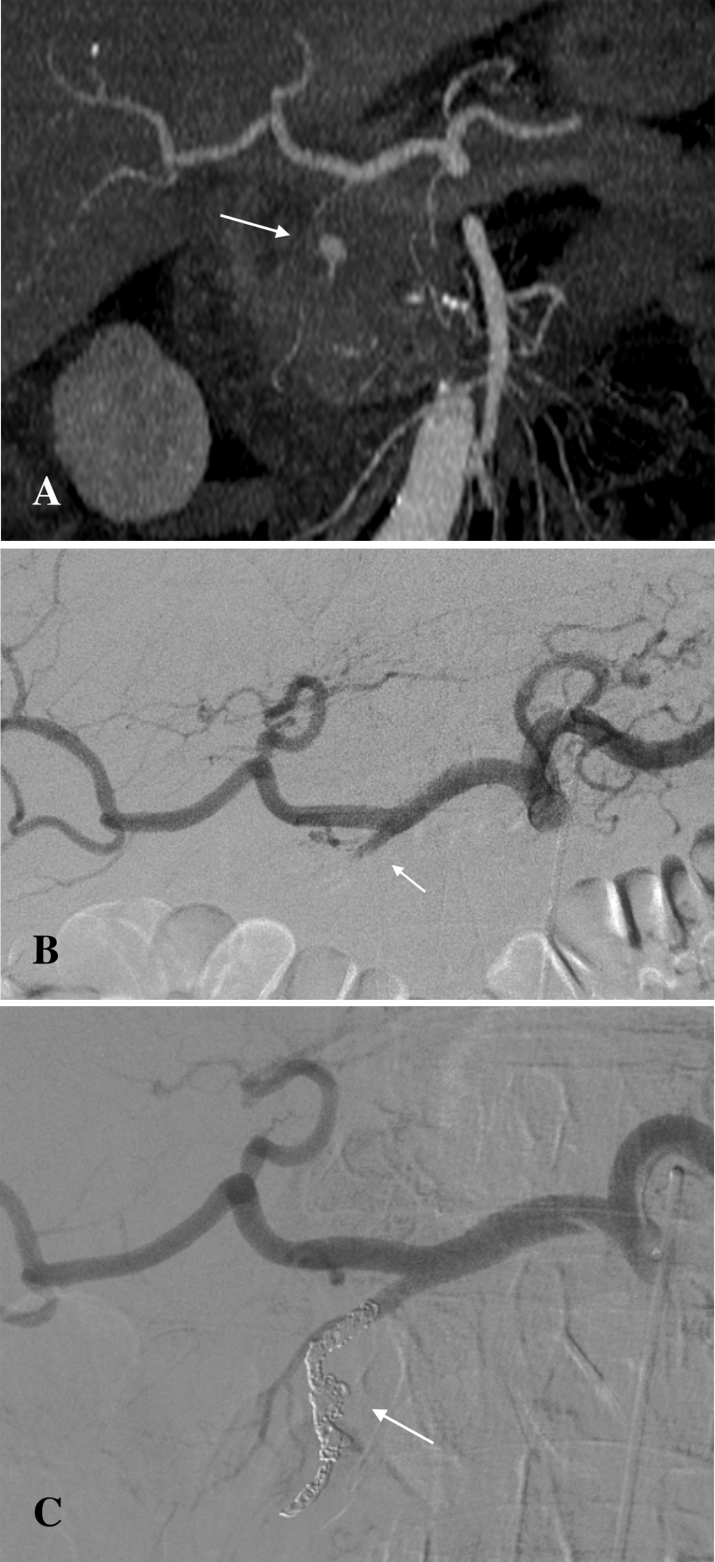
Pseudoaneurysm of the gastroduodenal artery pre- and post-coil embolization. (A) Pseudoaneurysm of the gastroduodenal artery visible on the contrast-enhanced CT (arrow). (B) Digital Subtraction Angiography (DSA) image showing vasospasm and thrombosis of the gastroduodenal artery with no active bleeding (arrow). (C) Post-embolisation DSA image showing successful coil embolisation of the gastroduodenal artery (arrow).
